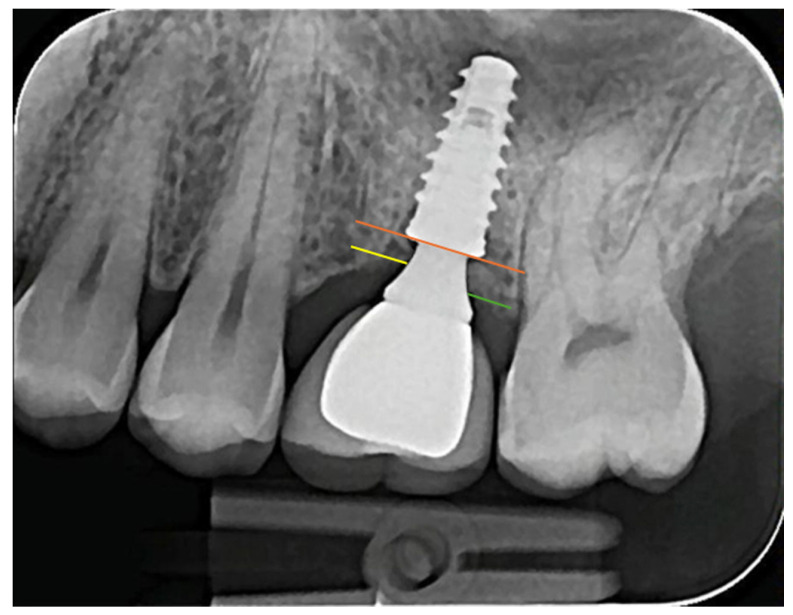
Representative image of the MBL measured in each radiography. The distance between the implant platform (orange line) to the first contact with the bone in the IA set in mesial (yellow line) represents the MBLm; in distal, the distance between the orange and green lines represents the MBLd.
